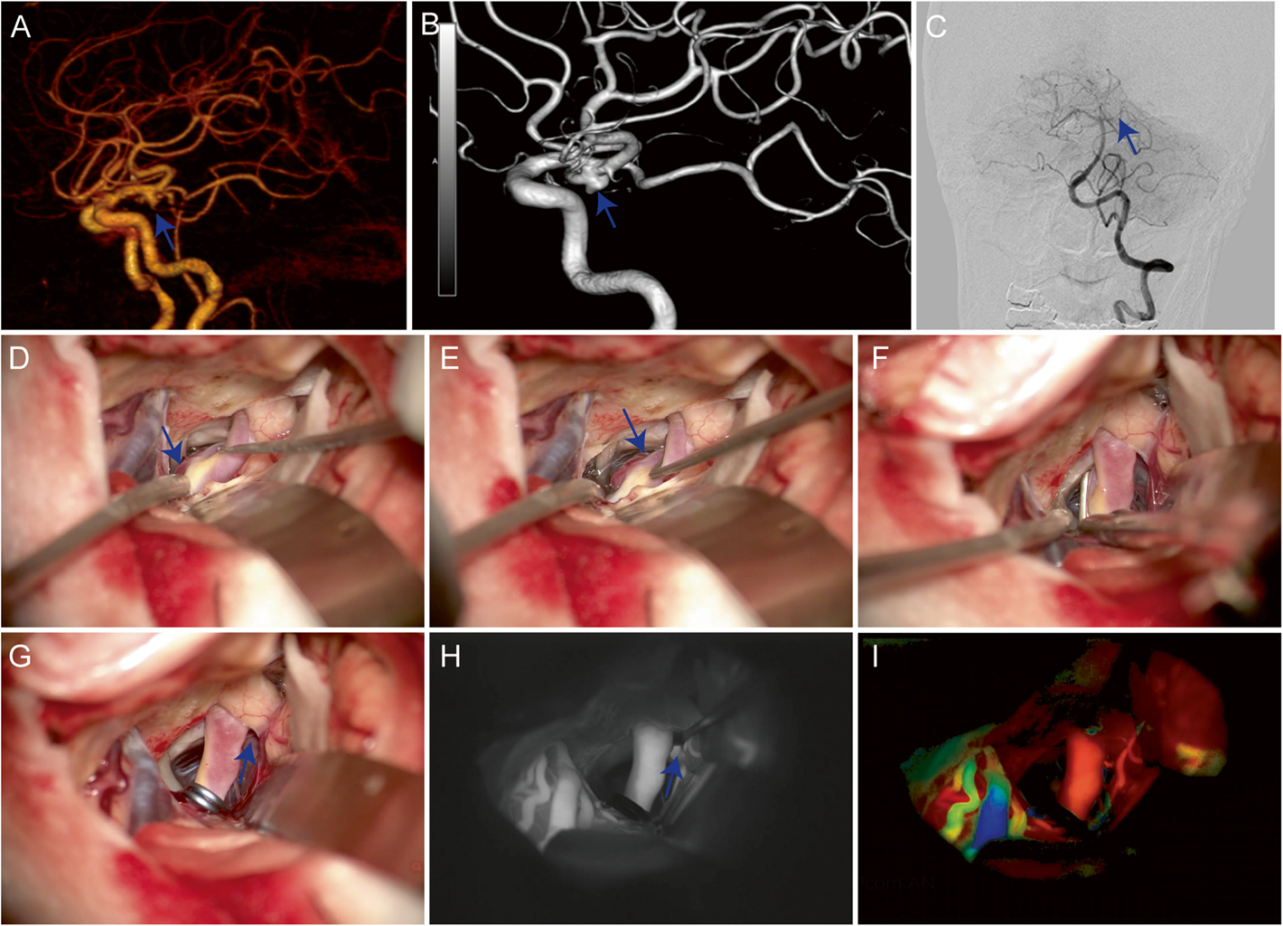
A left posterior communicating artery (PComA) aneurysm (4 × 3 mm, blue arrow) was diagnosed by computed tomography angiography (a), digital subtraction angiography (DSA) (b). DSA also indicated that the artery (blue arrow) was fetal-type posterior cerebral artery (c). During surgery, the lateral (d) and body (e) of aneurysm was exposed. The aneurysm neck was clipped with a cross-window aneurysm clip (f) and PComA (blue arrow) was visible (g). Conventional ICG-VA (h) and FLOW 800 analysis (i) showed clipping was satisfactory and the parent artery was unobstructed (blue arrow)
